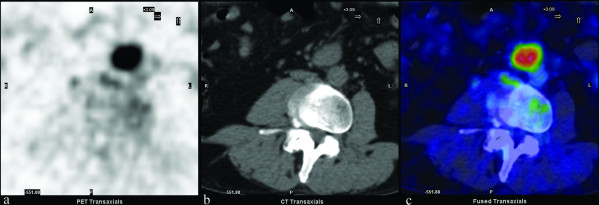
FDG-PET/CT transaxial views of the mesenterial lesion. (A) PET; (B) low-dose CT; (C) PET/CT fusion.
